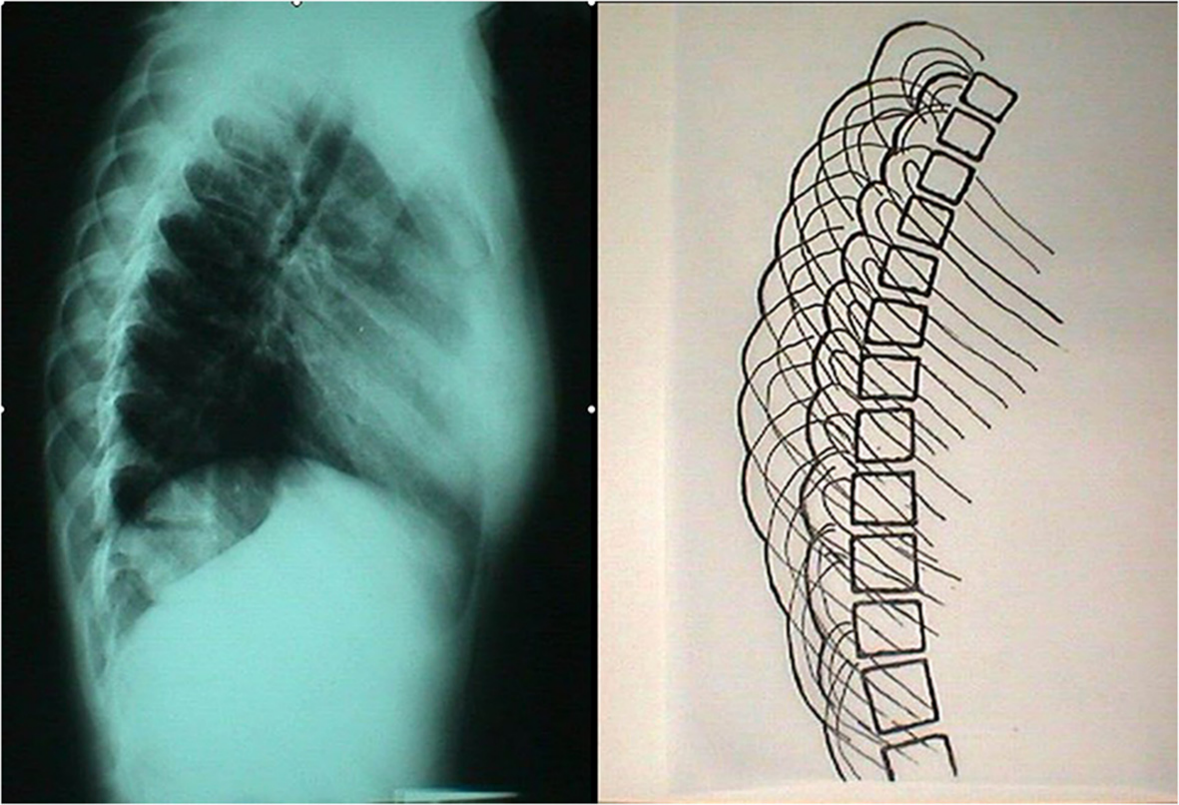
The double rib contour sign (DRCS) of the thoracic cage in a standing spinal radiograph.
